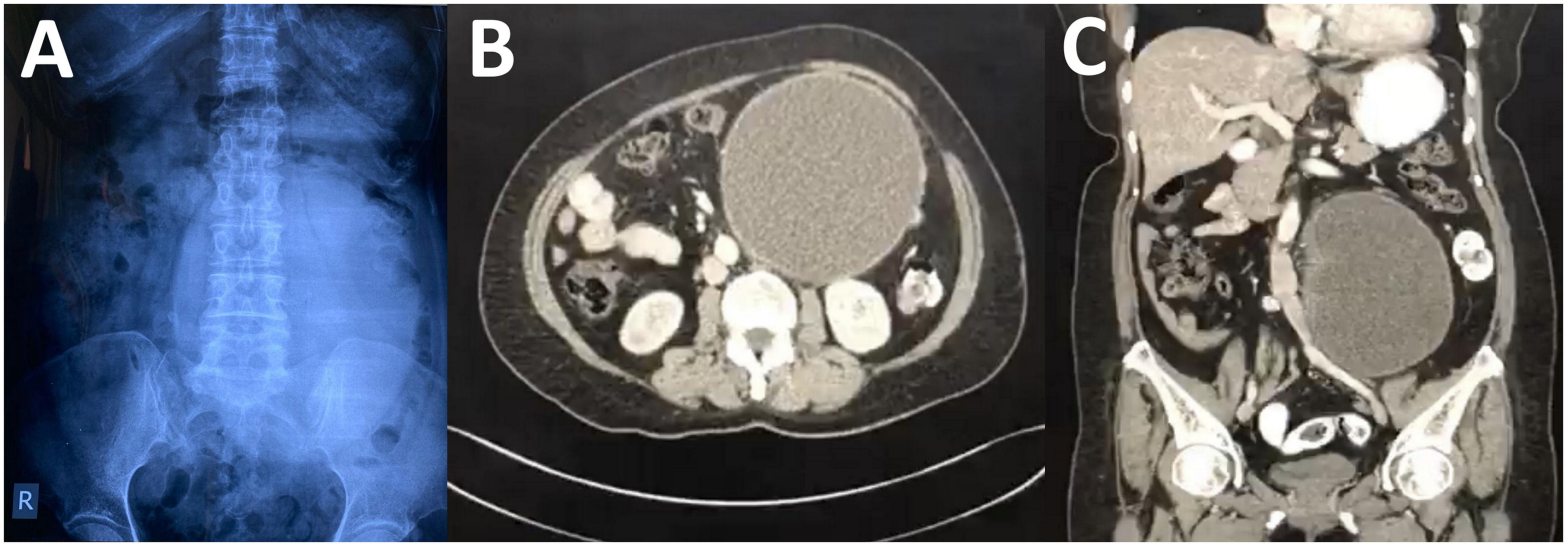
(A) Abdominal radiograph showing a huge oval radiopaque lesion on the left side. There was no dilated bowel visualized. (B) Contrast-enhanced computed tomography (CT) of the abdomen at axial phase showing a large well-defined homogenous lesion. (C) The homogenous lesion visualized at the coronal section.
